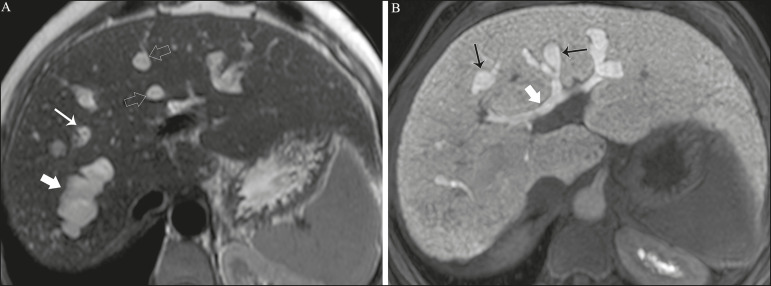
Axial T2-weighted MRI sequence (A) and axial T1-weighted MRI sequence in late hepatobiliary phase (B), showing dilatation of the intrahepatic bile duct (thick arrows) and sparse cysts (open arrows), some with a central dot of hypointense signal on T2 (thin white arrow), filled with the contrast agent in the late phase (black arrows), proving that there is communication with the bile duct, which is consistent with the diagnosis of Caroli disease.
